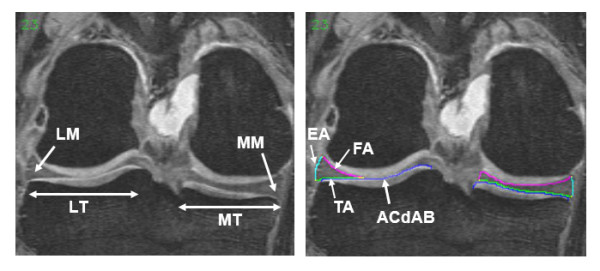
Coronal MR images of the right knee without segmentation (left) and with segmentation (right) of the medial (MM) and lateral meniscus (LM). The segmented surface of the tibial plateau area (ACdAB) of the medial (MT) and lateral tibia (LT) is shown in blue. The segmented surfaces of the meniscus are shown in green (tibial area = TA), magenta (femoral area = FA), and turquoise (external area = EA).
